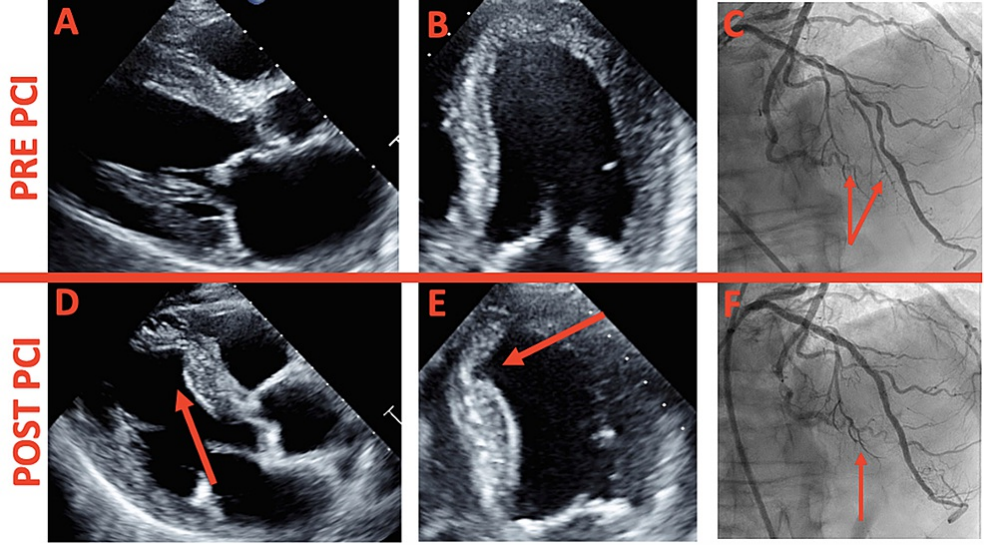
Echocardiography and coronary angiography. (A) Pre-PCI TTE parasternal long view with normal septal thickness. (B) Pre-PCI TTE apical view with normal apical and septal wall thickness. (C) Pre-PCI with the diffuse LAD lesion on coronary angiography measuring 80 mm with ostial stenosis of midseptal branch and diagonal branch (red arrows: two midseptales). (D) Post-PCI TTE parasternal long view with new septal thinning, but no perforation (red arrow: septal defect). (E) Post-PCI TTE apical view with same septal thinning redemonstrated (red arrow: septal defect). (F) Post-PCI of LAD with loss of midseptal branch (red arrow: only one septal left). PCI: percutaneous coronary intervention, TTE: transthoracic echocardiography, LAD: left anterior descending coronary artery.
